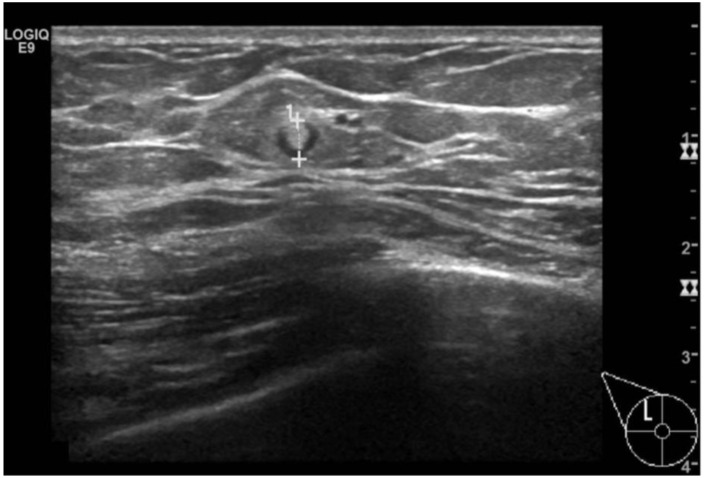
An intra-mammary lymph-node in the upper-outer quadrant of the right breast of 70-year-old men. B-mode ultrasound shows the small reniform mass with a central hyperechoic fatty hilum and the hypoechoic cortex.
